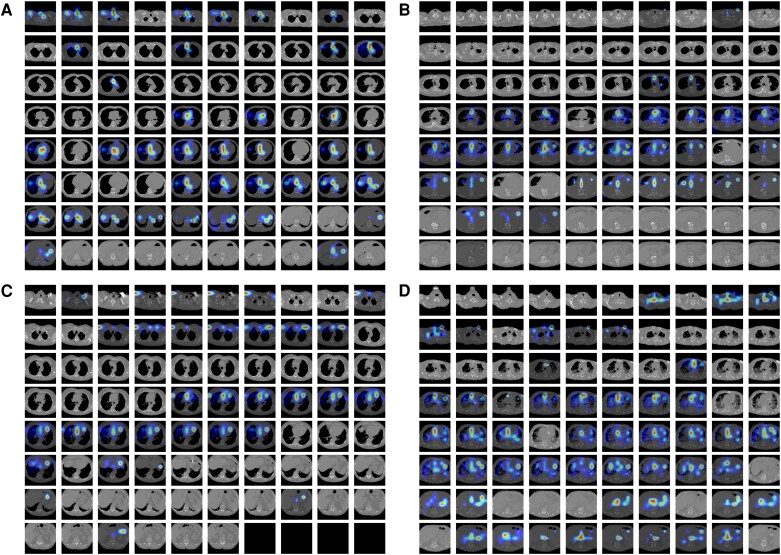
Model interpretability. Sample visualizations of Gradient-weighted Class Activation Mapping saliency maps overlaid on axial-oriented computed tomography scans for four patients with abnormal left-ventricle ejection fraction. These maps highlight regions within the computed tomography scans that contributed most significantly to the artificial intelligence model’s predictions, providing insights into the features used for classification. (*A*) An enlarged heart and dilated superior vena cava reflecting increased venous pressure (due to heart not working hard enough to keep up with venous return. (*B*) Ascending aorta—especially when calcified. (*C*) Presence of pacemakers and pacemaker wires. (*D*) Pulmonary infiltrates from oedema.
